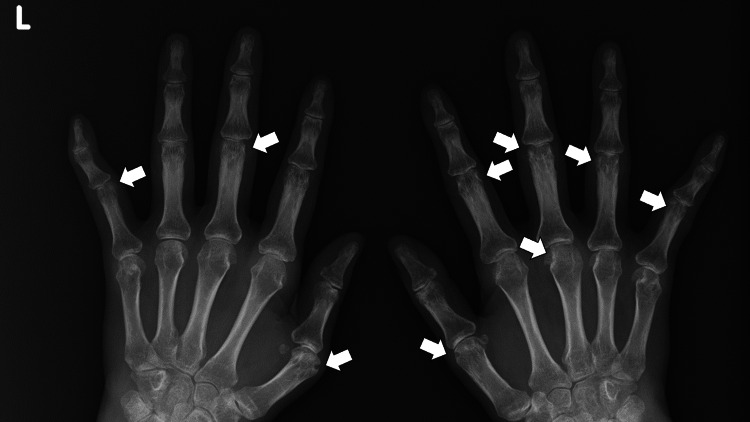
X-ray of bilateral hands showing multiple bone erosions.
